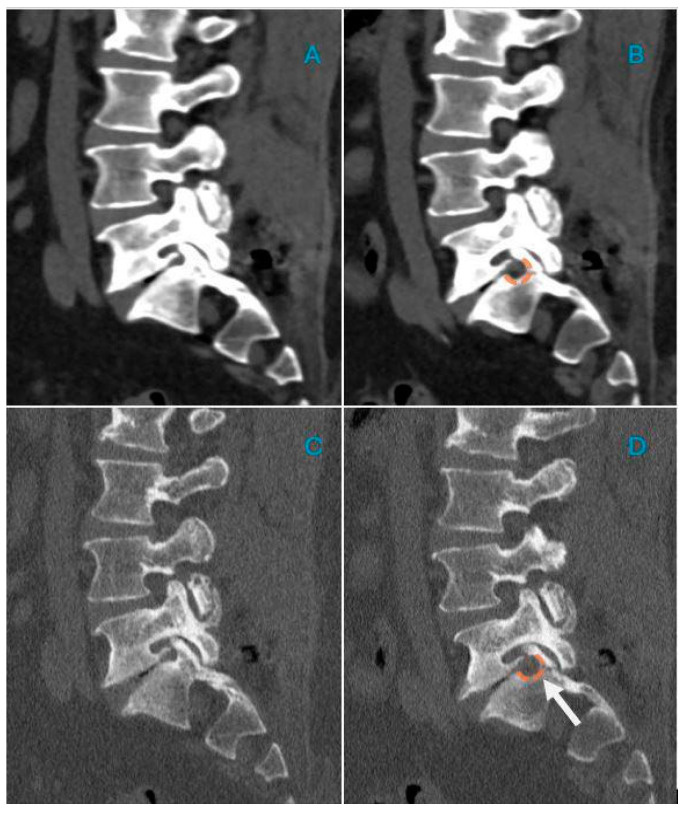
CT sagittal reconstructions for soft tissues (A,B) and bone (C,D); a 68-year-old patient presenting with lumbar sciatica. Preoperative images (A,C) show stenosis of the right L5–S1 foramen, with associated osteophytic formations. Postoperative findings reveal widening of the foramen floor (orange dashed line, images (B,D)), with persistence of osteophytic spurring, but with an enlargement that mainly involves the inferior and posterior sectors of the foramen (white arrow in (D)).
